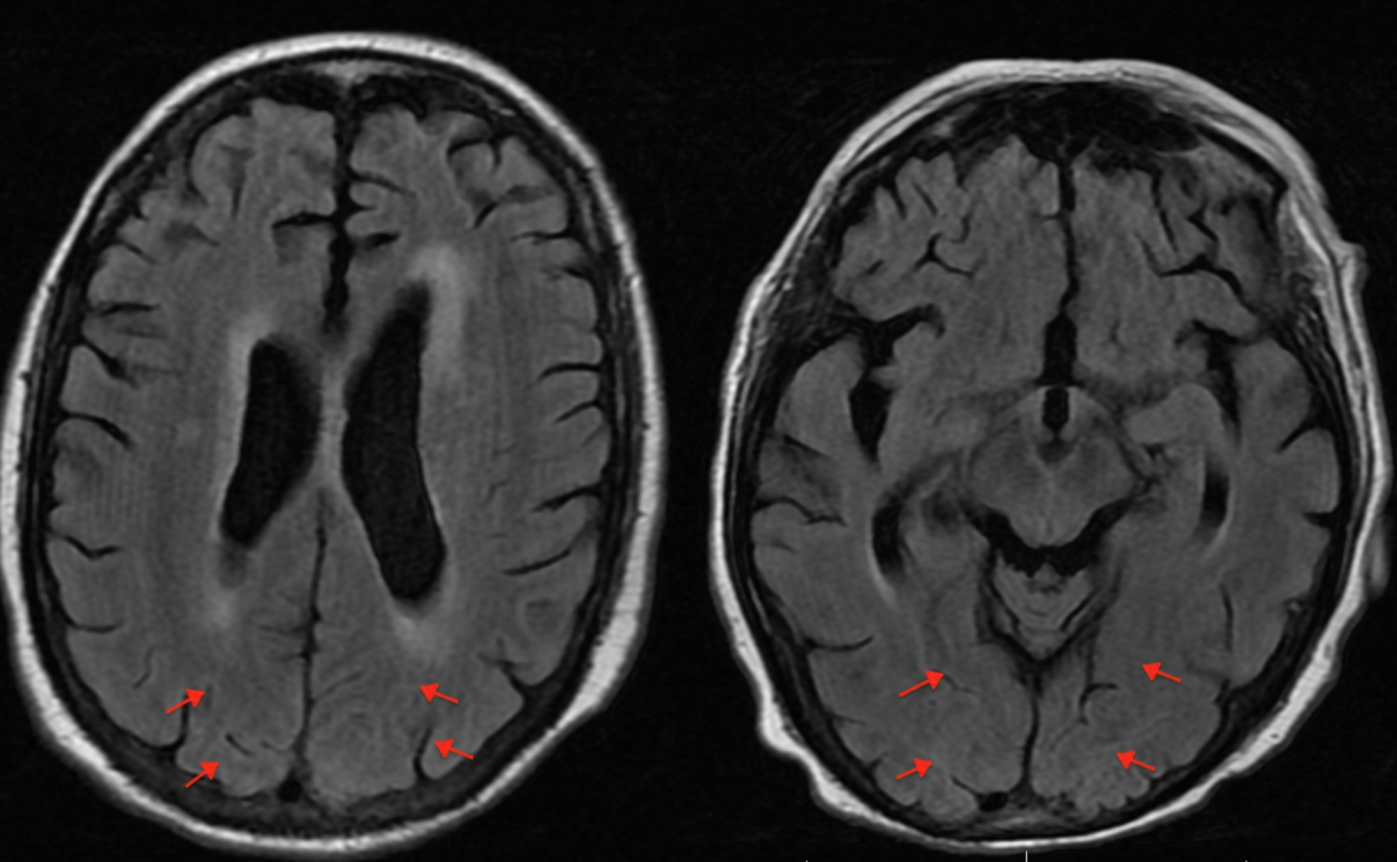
No SAH seen on MRI brain. SAH, subarachnoid hemorrhage
